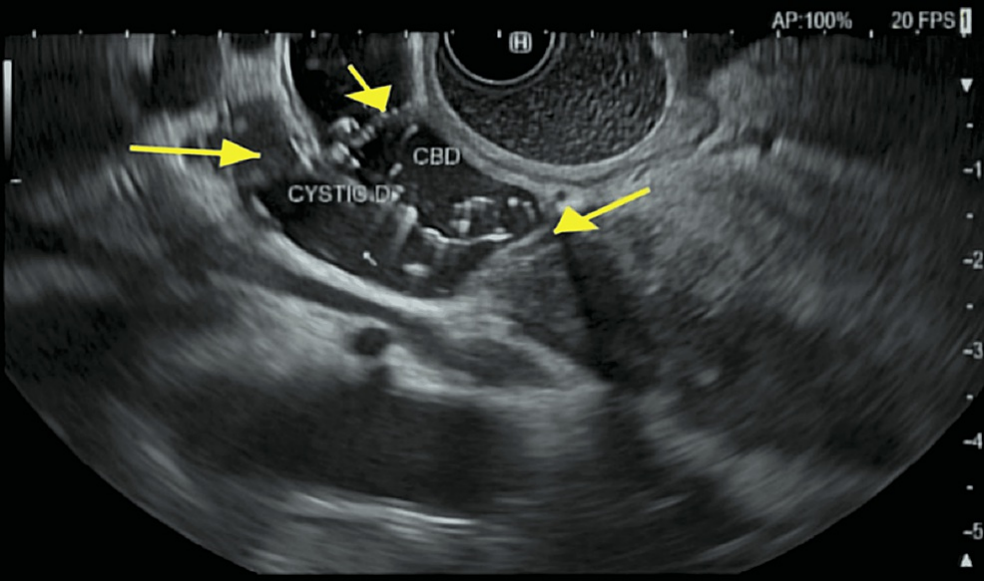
There was dilation in the common bile duct and the cystic duct, which measured 12 mm. The cut-off is right at the beginning of its intrapancreatic portion. Extensive hyperechoic material consistent with sludge was visualized endosonographically in the common bile duct, the cystic duct, and the gallbladder. The peri-ampullary portion of the biliary duct and the pancreatic duct was intact, without dilation.
